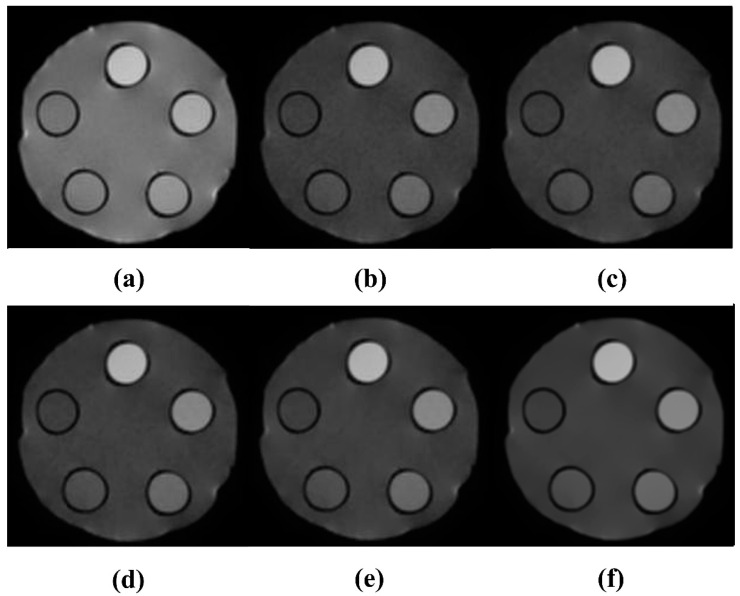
Optimization of the smoothing factor in b = 1000 s/mm^2^ diffusion-weighted magnetic resonance images: (a) 0.001, (b) 0.013, (c) 0.040, (d) 0.080, (e) 0.120, (f) 0.150.
